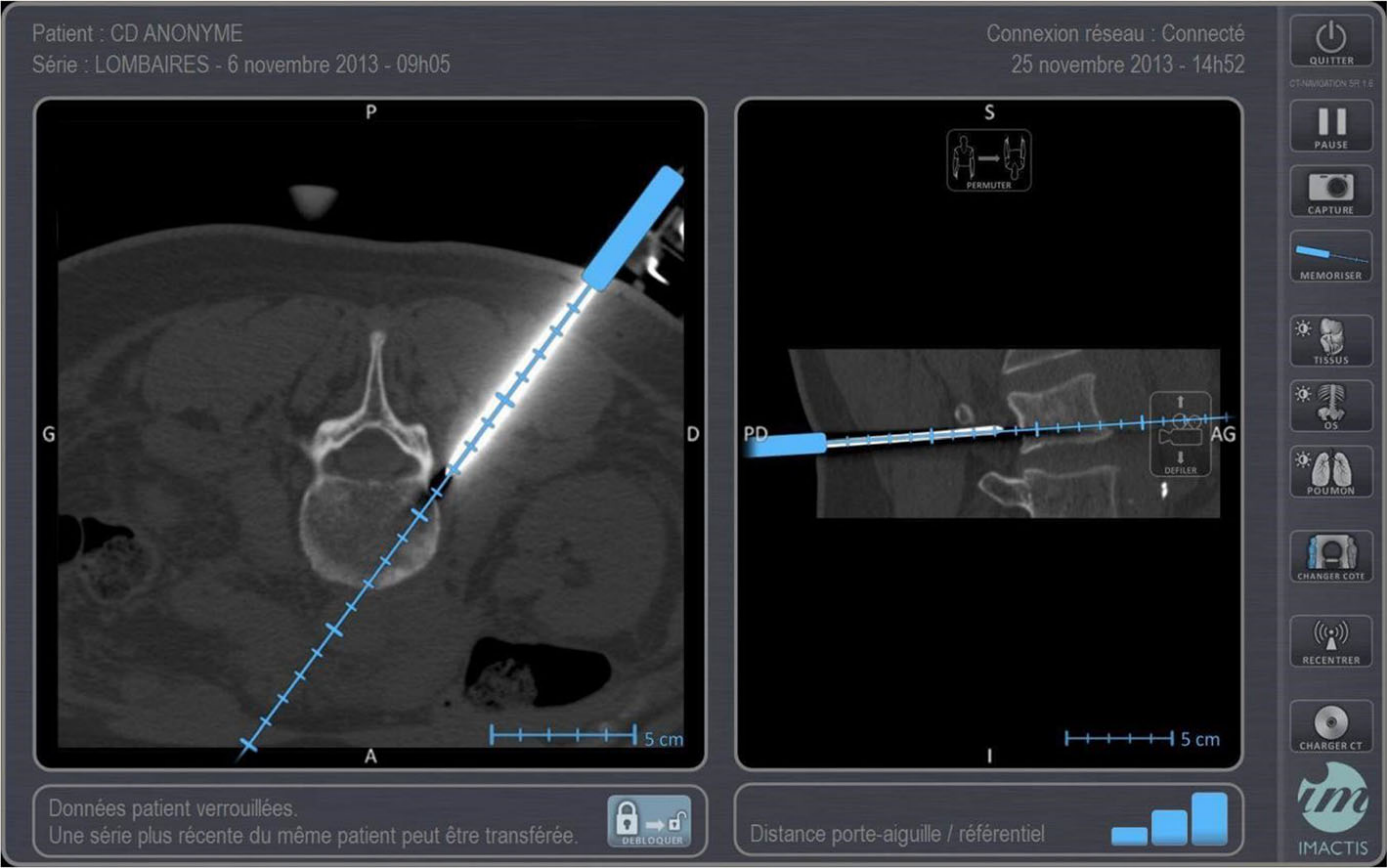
Screenshot of a computed tomography-guided percutaneous biopsy of a spinal lesion. The biopsy has been carried out using navigation assistance provided by the IMACTIS-CT® system. It can be seen that the estimated position of the trocar (*blue*) is accurate with respect to the real position of the trocar (*white*)
